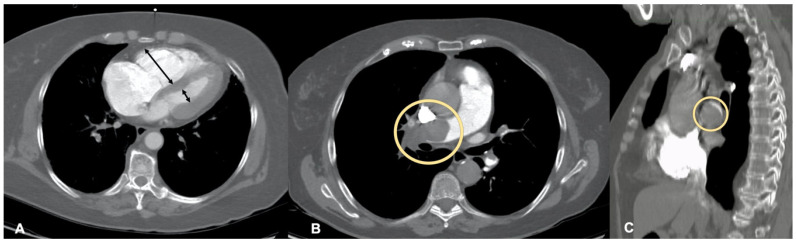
(A) Axial CT pulmonary angiography image showing right ventricular dilatation with an RV/LV ratio > 1 (4.56/1.49 cm = 3.06; black arrows), consistent with right ventricular strain. (B,C) Axial and sagittal images demonstrating a thrombus located in the right main pulmonary artery, occupying >50% of the vessel lumen (yellow circles). Findings correspond to GmS3. RV/LV = right ventricle to left ventricle diameter ratio.
